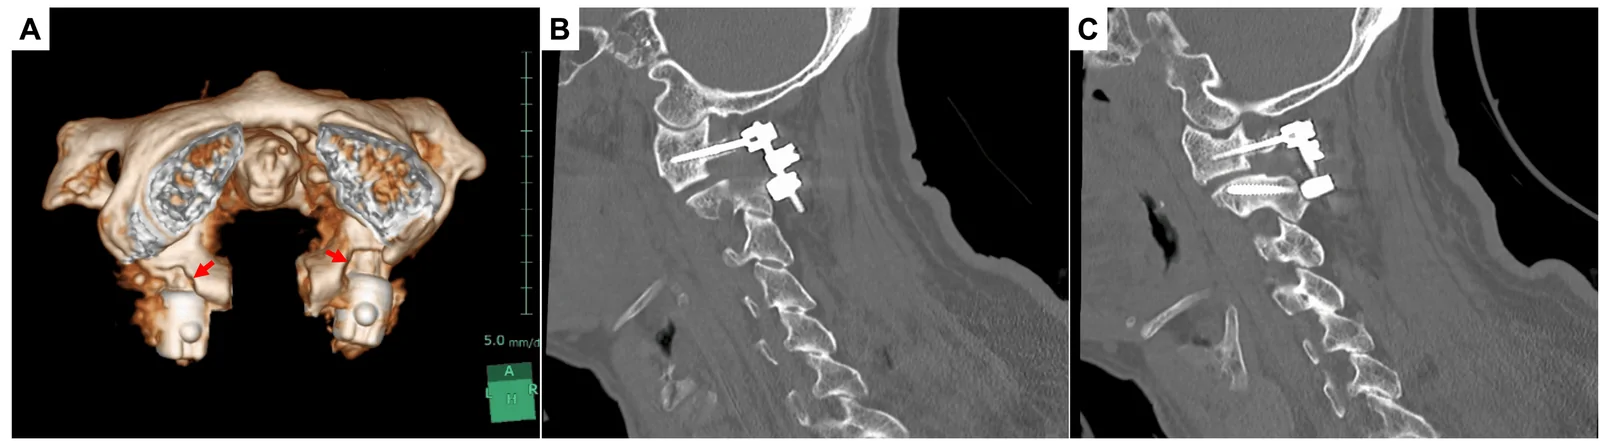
Postoperative CT demonstrating screw placement and the unroofing. The cranial aspects of the posterior arch are exposed by unroofing (A, red arrows). C1 LMSs are correctly placed (B, right side; C, left side). Abbreviations: CT, computed tomography; LMS, lateral mass screw.
